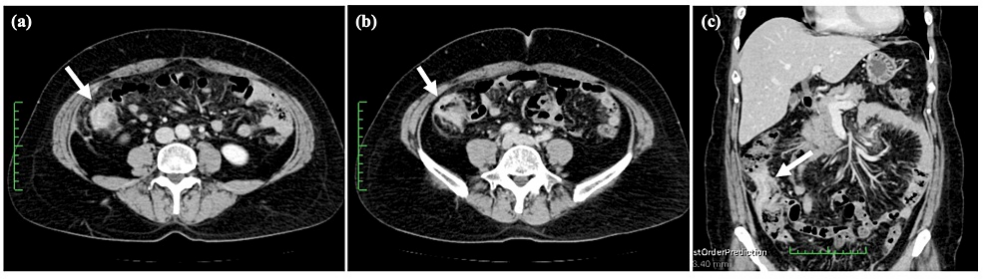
Abdominal CT images at diagnosis. Abdominal CT (a, b, c) shows the pronounced thickening of the bowel wall in the ascending colon (indicated by the arrow).
